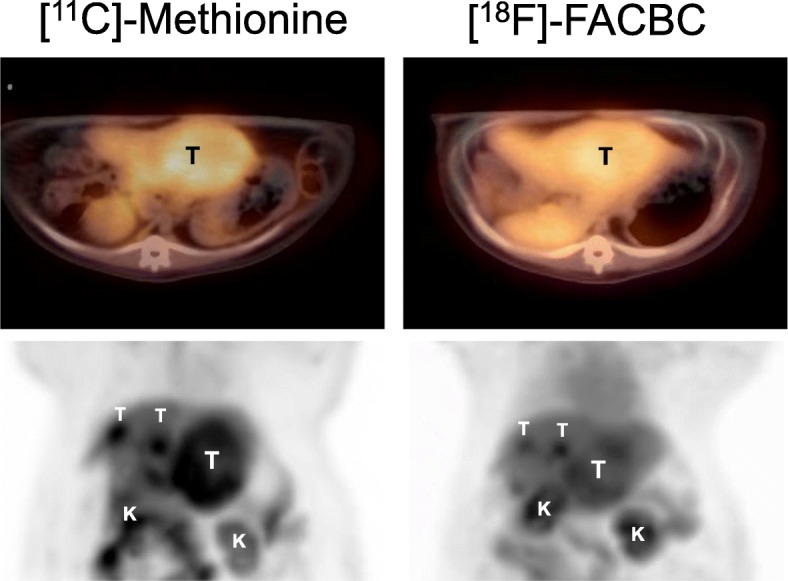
Comparison between [^11^C]Met and [^18^F]FACBC for PET imaging of HCC taken between 15 and 20 min post-injection. Top: PET/CT overlay (axial view). Bottom: maximum intensity projection (MIP, coronal view). T tumor (the large tumor in one lobe, also shown on the axial view, and two smaller tumors in a lobe behind); K kidney
